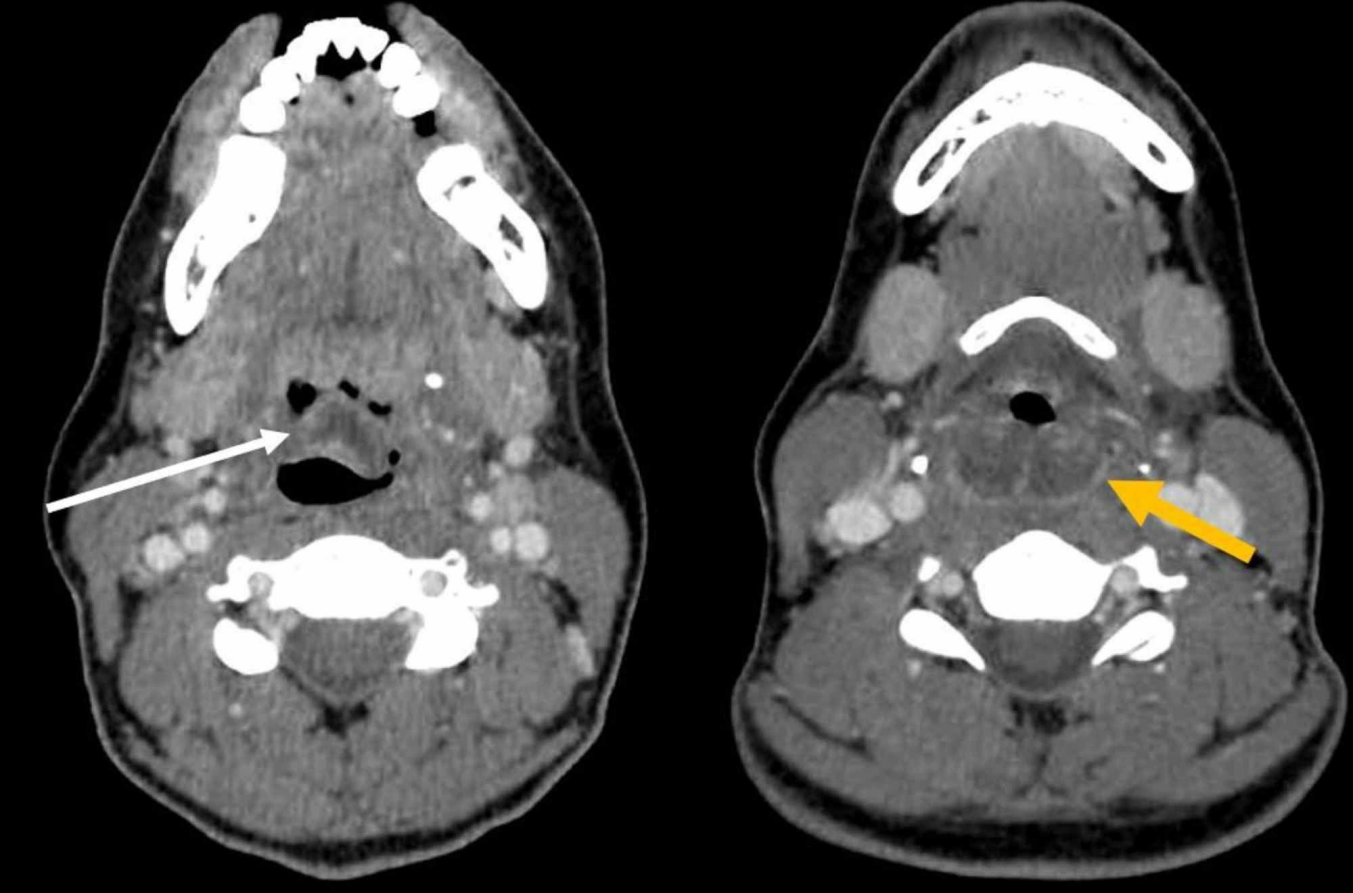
Axial computed tomography scans Axial computed tomography scans showing edema of the epiglottis (white arrow) and narrowing of the airway secondary to abscess and edema at the level of the hypopharynx (yellow arrow).
